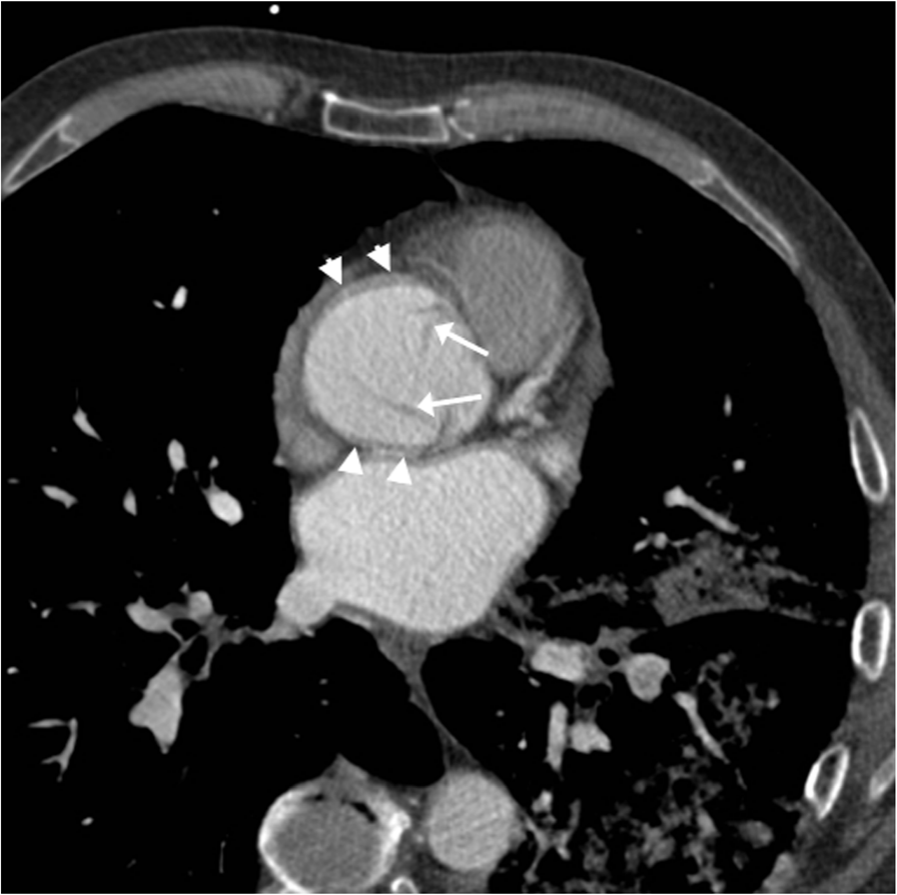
Initial gated non-cardiac computed tomographic angiogram. A gated non-cardiac axial contrast-enhanced computed tomographic scan of the sinuses of Valsalva is shown. Aortic motion artefact blurs the aortic wall anteriorly and posteriorly (arrowheads). This is the plane of aortic translocation during the cardiac cycle. The lines within the sinuses (arrows) were misinterpreted as aortic valve leaflets but lie above the cranial limit of normal leaflet tips.
